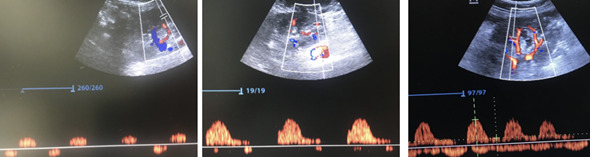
Case 2. Evolution of Doppler interlobar point of care ultrasound (POCUS), arterial flow over the baseline and venous flow under the baseline. In A, RRI 1 with venous flow alone in the diastole (maximum venous congestion). In B after a negative balance of 5 kg on dialysis a better arterial flow and moderate venous congestion are seen (flow in both times). In C, RRI 0.75 with normal venous flow after a negative balance of 10 kg on dialysis.
